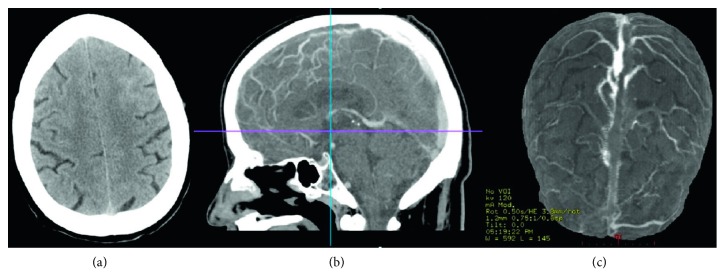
Brain computed tomography images. (a) Initial CT scan reveals loss of cortical-subcortical discrimination in the left frontal lobe and a local linear hyperdensity indicating subarachnoid haemorrhage. (b) CT venography: sagittal cut showing absence of permeability of the anterior two-thirds of the superior longitudinal sinus consistent with thrombosis. (c) 3D reconstruction of the CT venography.
